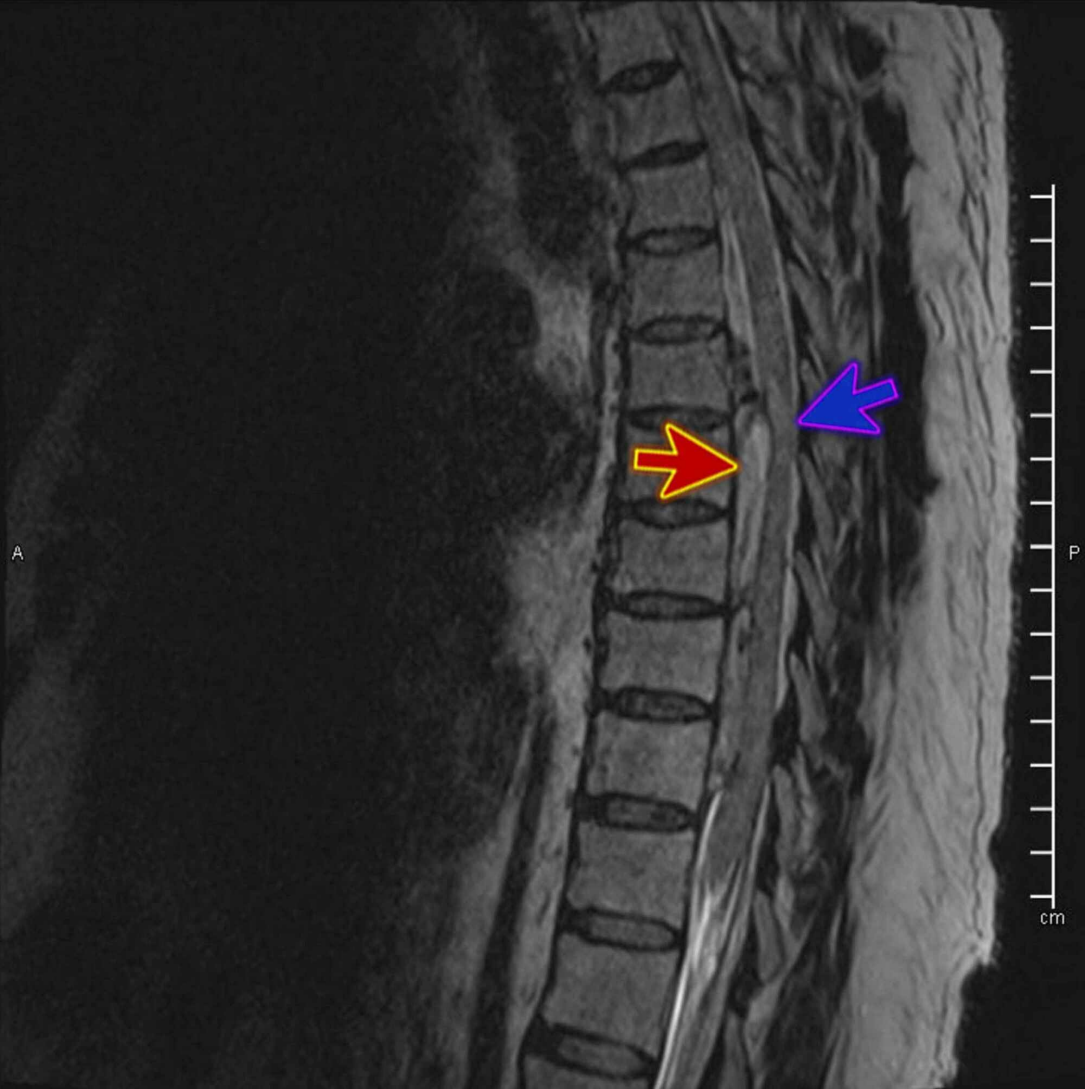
MRI T2WI sagittal view shows extensive extradural hematoma severe mass effect on the cord (red arrow) with evidence of edema (blue arrow), most severe at the level from the seventh to the tenth dorsal vertebra (D7-D10) MRI: magnetic resonance imaging, T2WI: T2-weighted image.
